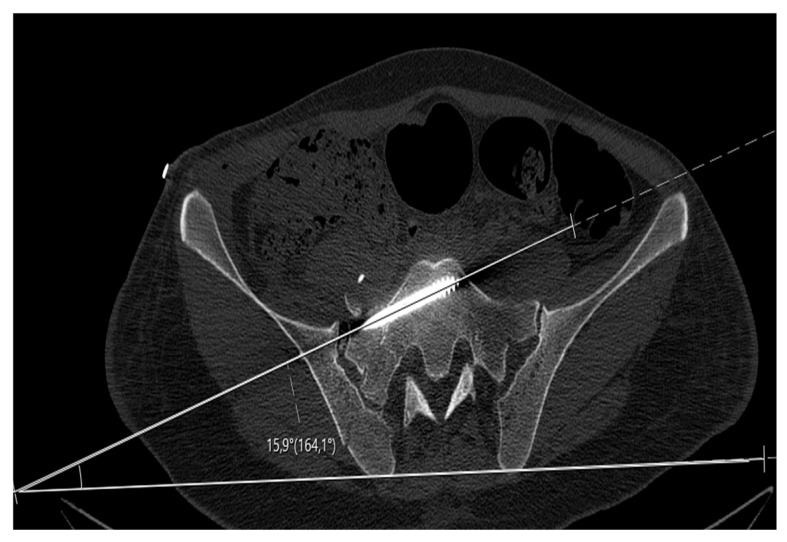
Postoperative measurement of the S1 screw anteversion angle on axial CT.
